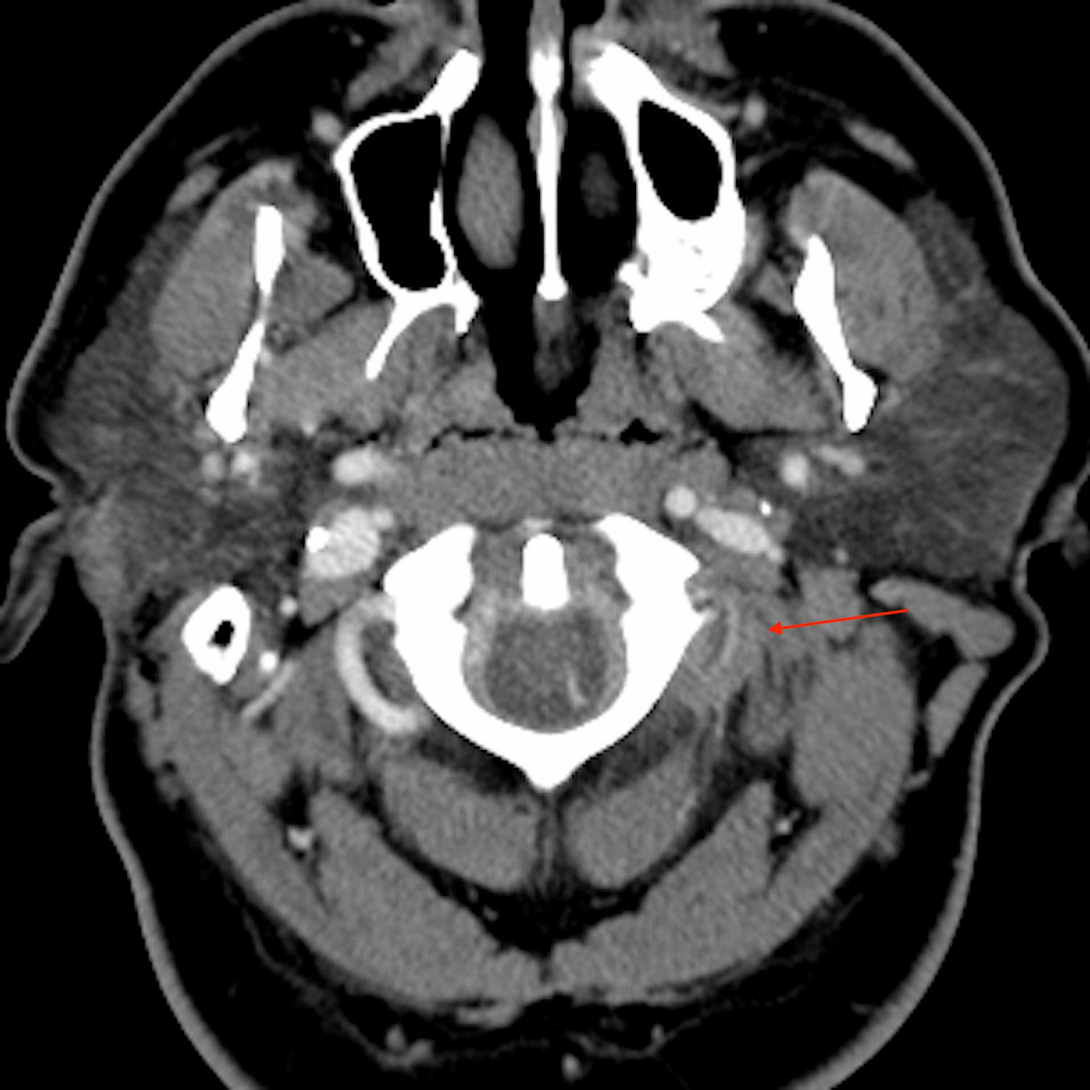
Axial-view contrast-enhanced neck CT performed as the initial imaging exam shows narrowed V3 segment with surrounding isodense hematoma.
